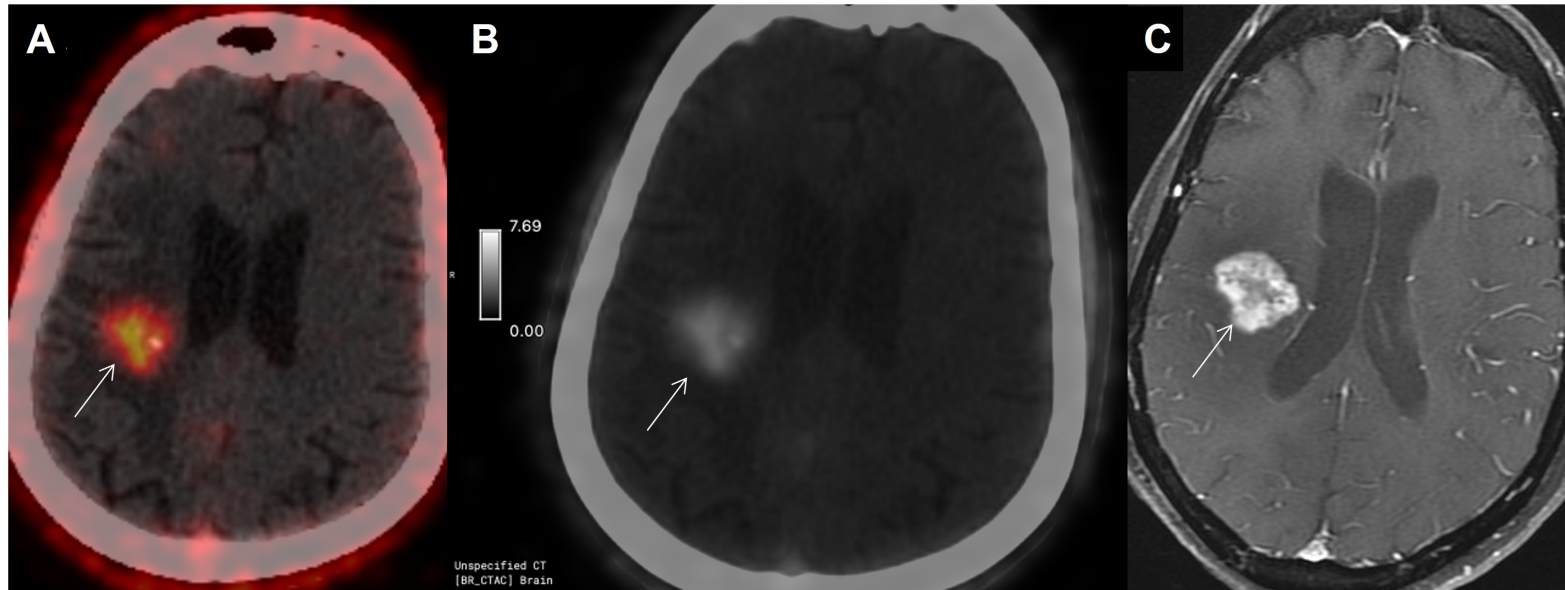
52-year-old case (patient 4), operated case of Grade IV GBM, post radiotherapy and temozolamide, index tumor was in left temporal region in November 2020. Now presented with left sided weakness. Imaging as advised with a clinico-radiological suspicion of post-treatment changes in the low-dose radiotherapy region in the opposite hemisphere. High intensity FAPI uptake was seen in right hippocampal region (more than background) on axial gray scale PET image (A, arrow), with SUV_max_ of 3.2; which matched the uptake on axial FET PET/CT (B, arrow) with T-W ratio of 3.6, suggestive of recurrence. Uptake pattern was concordant with enhancing lesion on axial post-contrast T1-weighted MR sequence (C, arrow) which was reported as BT-RADS 3B. Patient showed clinical worsening within 3 months and died. FET: fluoro-ethyl tyrosine; GBM: glioblastoma
